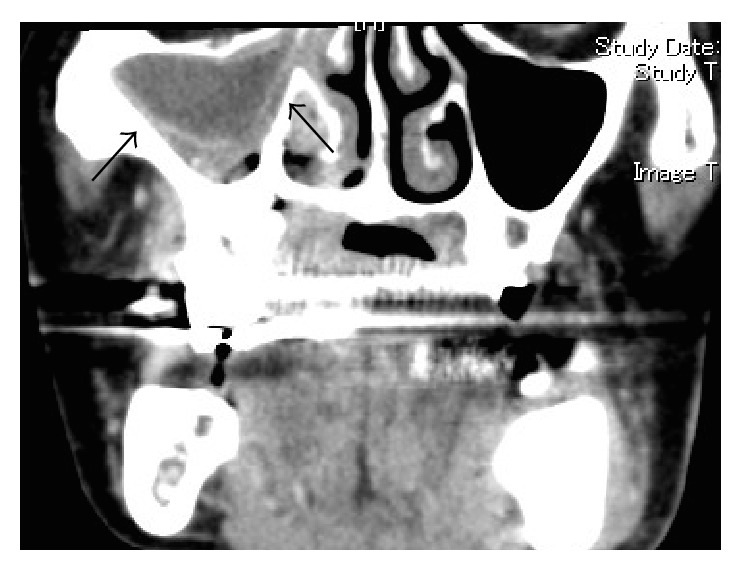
Preoperative CT revealing an abscess formation.
